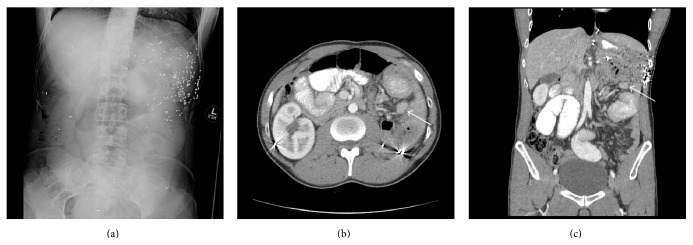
Abdominal X-ray shown in (a), depicting the number of shrapnel left in the left side of the body after the gunshot injury, in addition to dilated loops of bowel. CT scan with oral and intravenous contrast shows the splenosis deposit (white arrow) in axial (b) and coronal (c) views.
